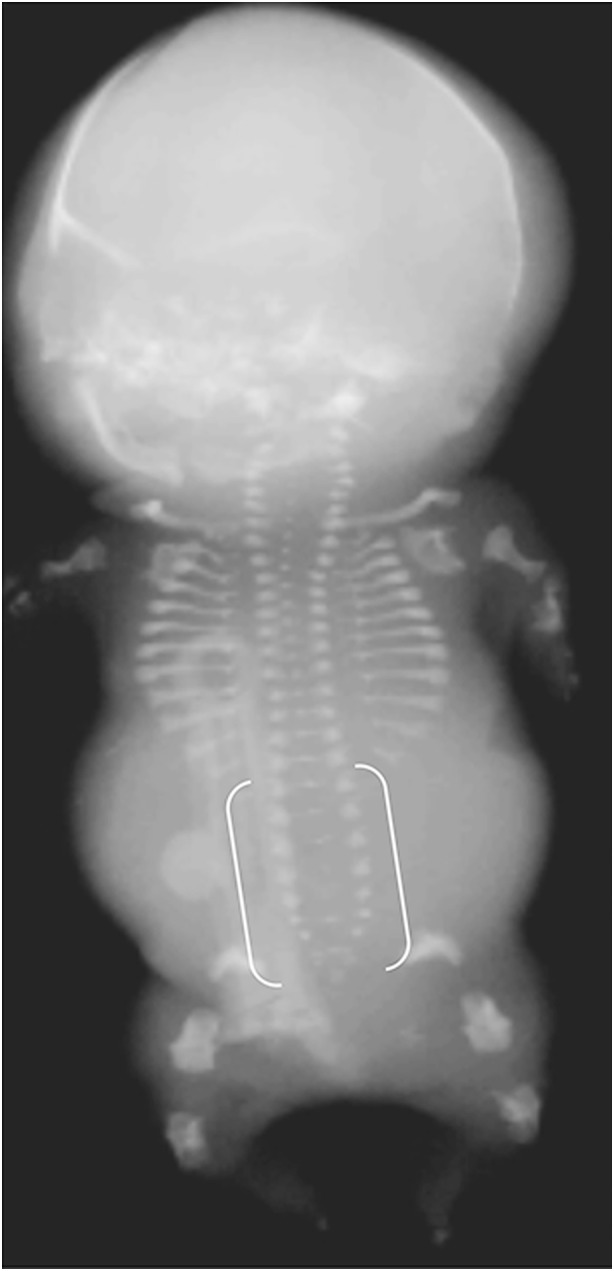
Radiographic features in a female fetus with achondrogenesis type IB*.* Anteroposterior radiograph shows short ribs, short limbs, deficient ossification of the ischia and widening of the lumbar interpedicular distances (*brackets*), which has been likened to the shape of a cobra’s head. [Image reproduced with permission from Radcliffe Publishing [38], page 106, case 3]
